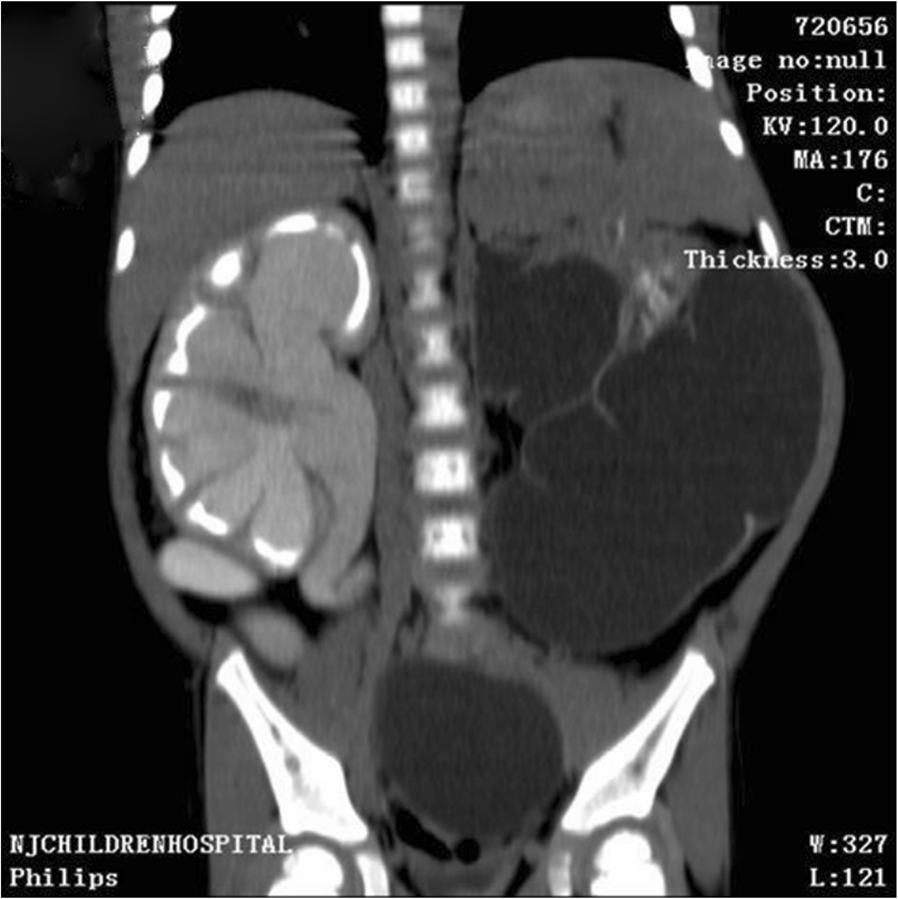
Contrast-enhanced computed tomography scan shows renal dysplasia with giant ureter on the left side and right hydronephrosis with the whole right ureter dilatation
